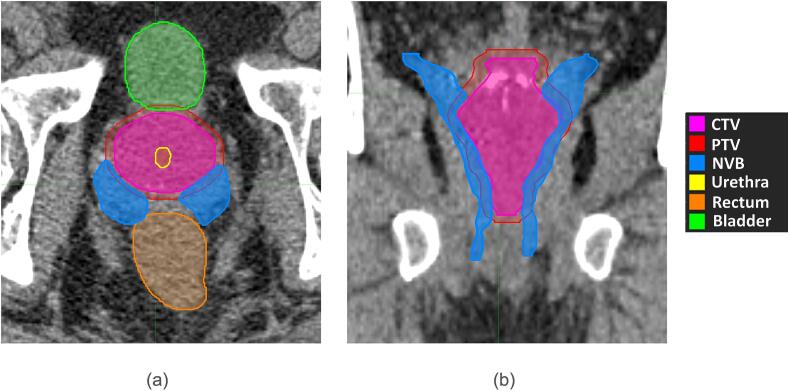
(a) axial and (b) coronal CT slice of a patient with the delineated CTV, PTV, NVB bundles and surrounding OARs.
